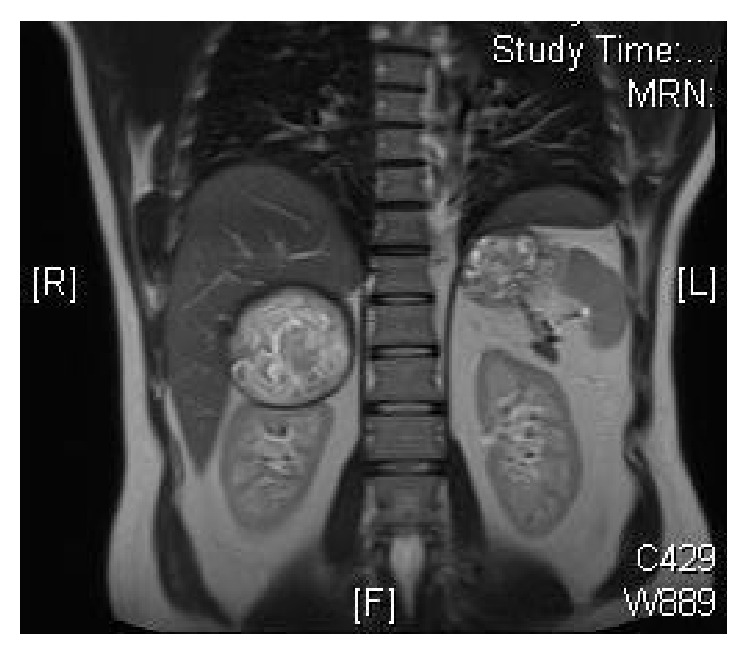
MRI with R adrenal mass.
